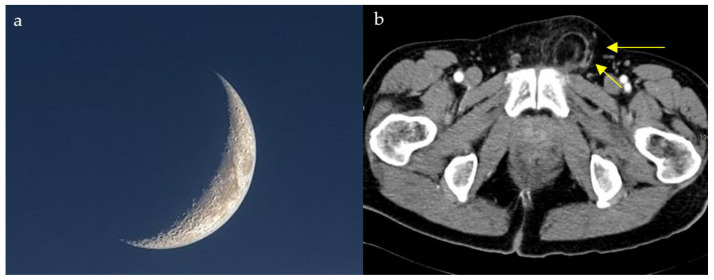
Compression and lateral displacement of the inguinal canal contents by the hernia to form a semicircle of tissue seen lateral to the hernia on axial CT images of the pelvis (yellow arrows) (b), resembling a crescent moon (a).
